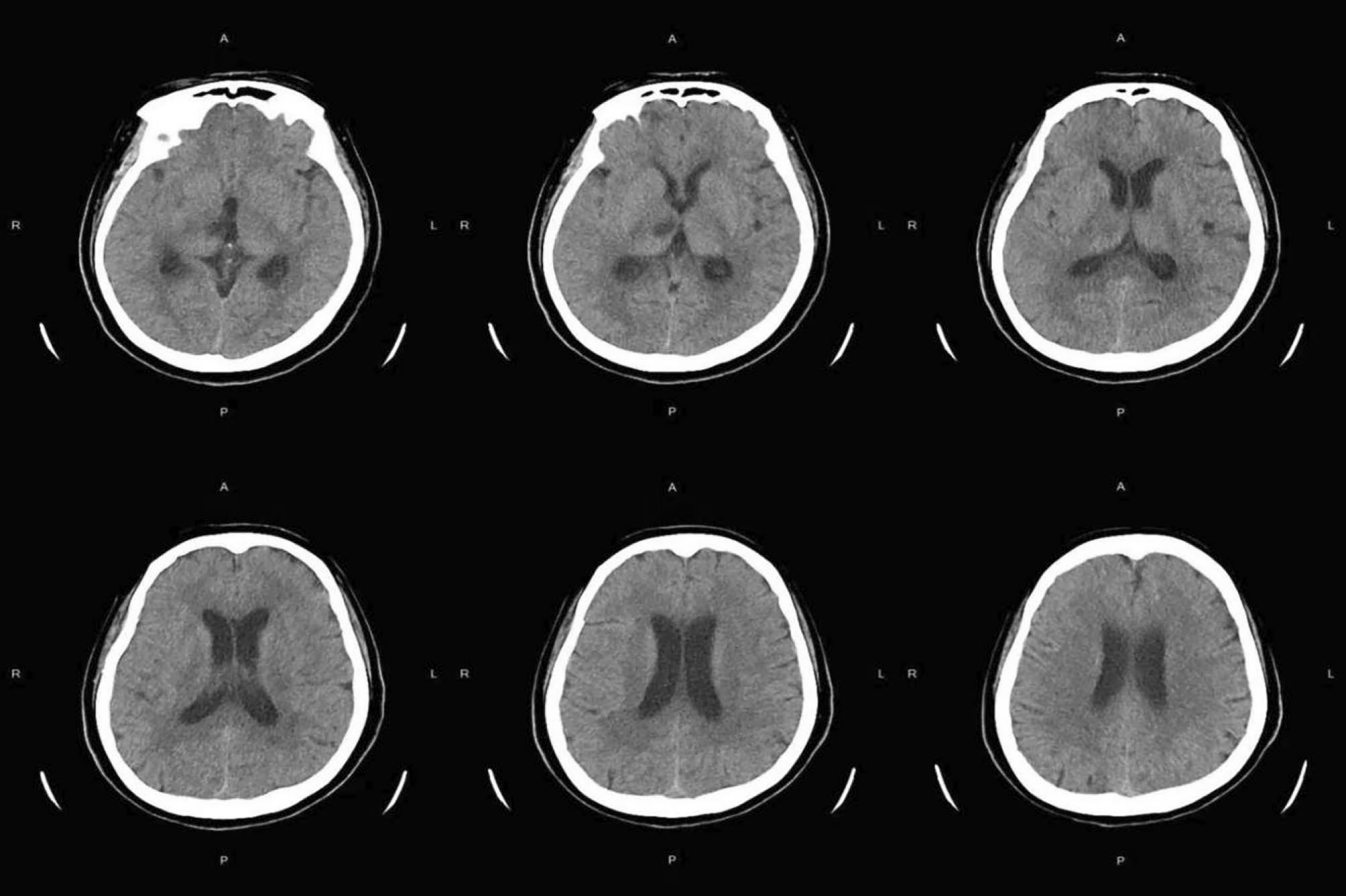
Brain computed tomography scanning
